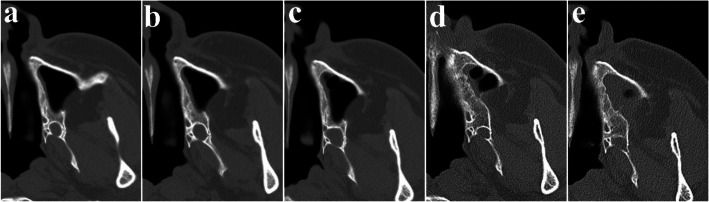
CT follow-up showing the slow growth rate of recurrent odontogenic keratocyst (OKC). a 3 years after the first surgery (maximum diameter: 7.76 mm), (b) 4 years after the first surgery (9.07 mm), (c) 5 years and 2 months after the first surgery (10.81 mm), (d) 3 years and 4 months after the second surgery (7.35 mm), (e) 4 years and 4 months after the second surgery (8.37 mm)
